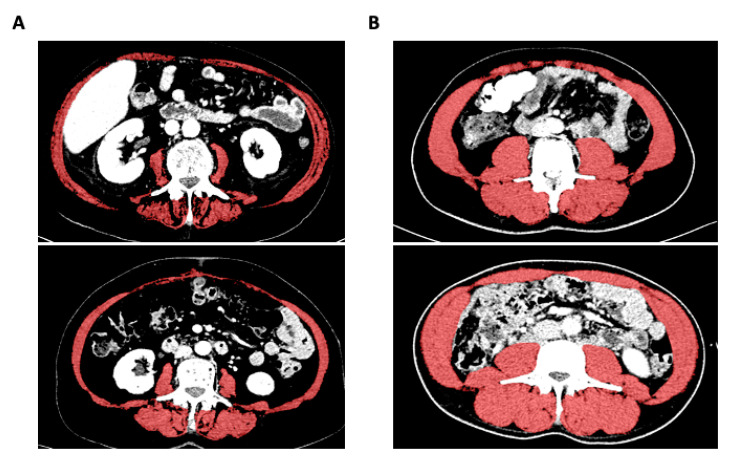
Evaluation of the L3SMI using the 3D slicer tool. Exemplary presentation of CT scans from patients with low (A) or high (B) L3SMI value.
